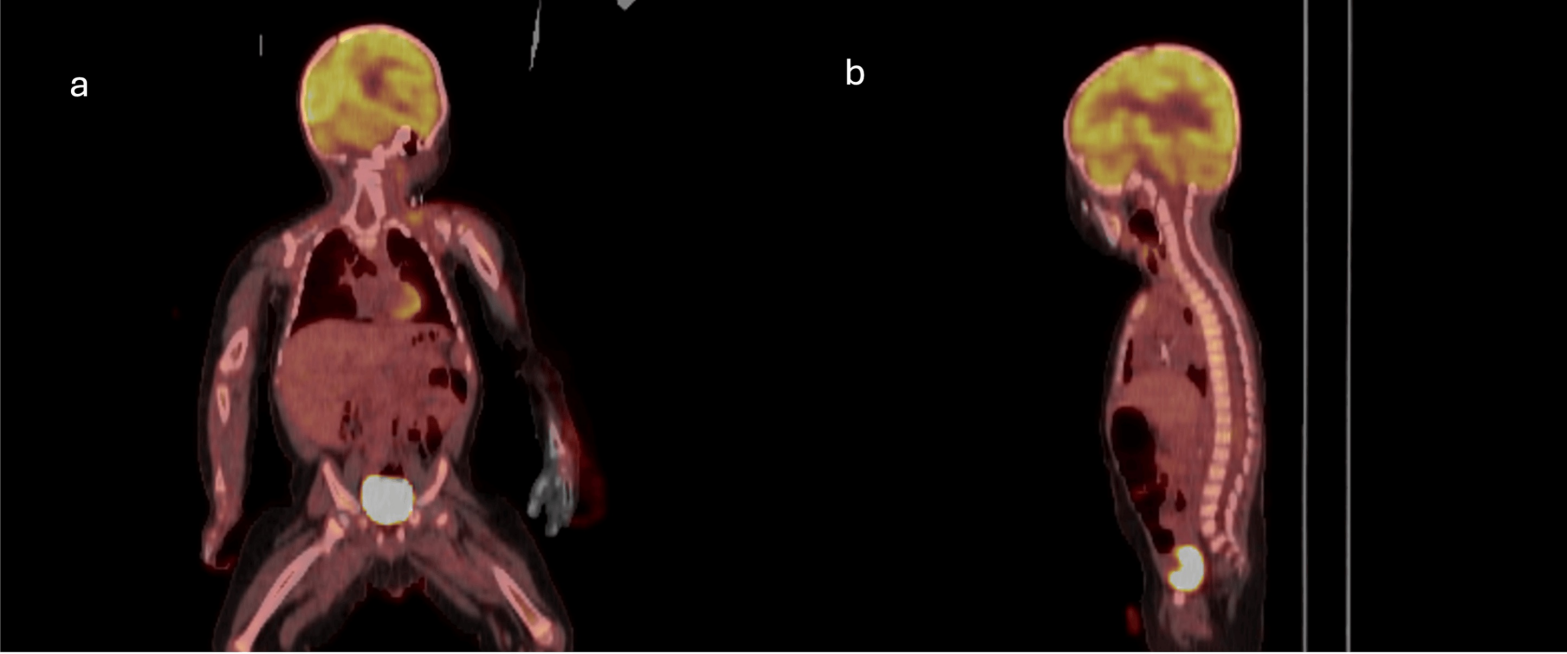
Coronal (a) and Sagittal (b) PET/CT demonstrates no distant metastasis.
